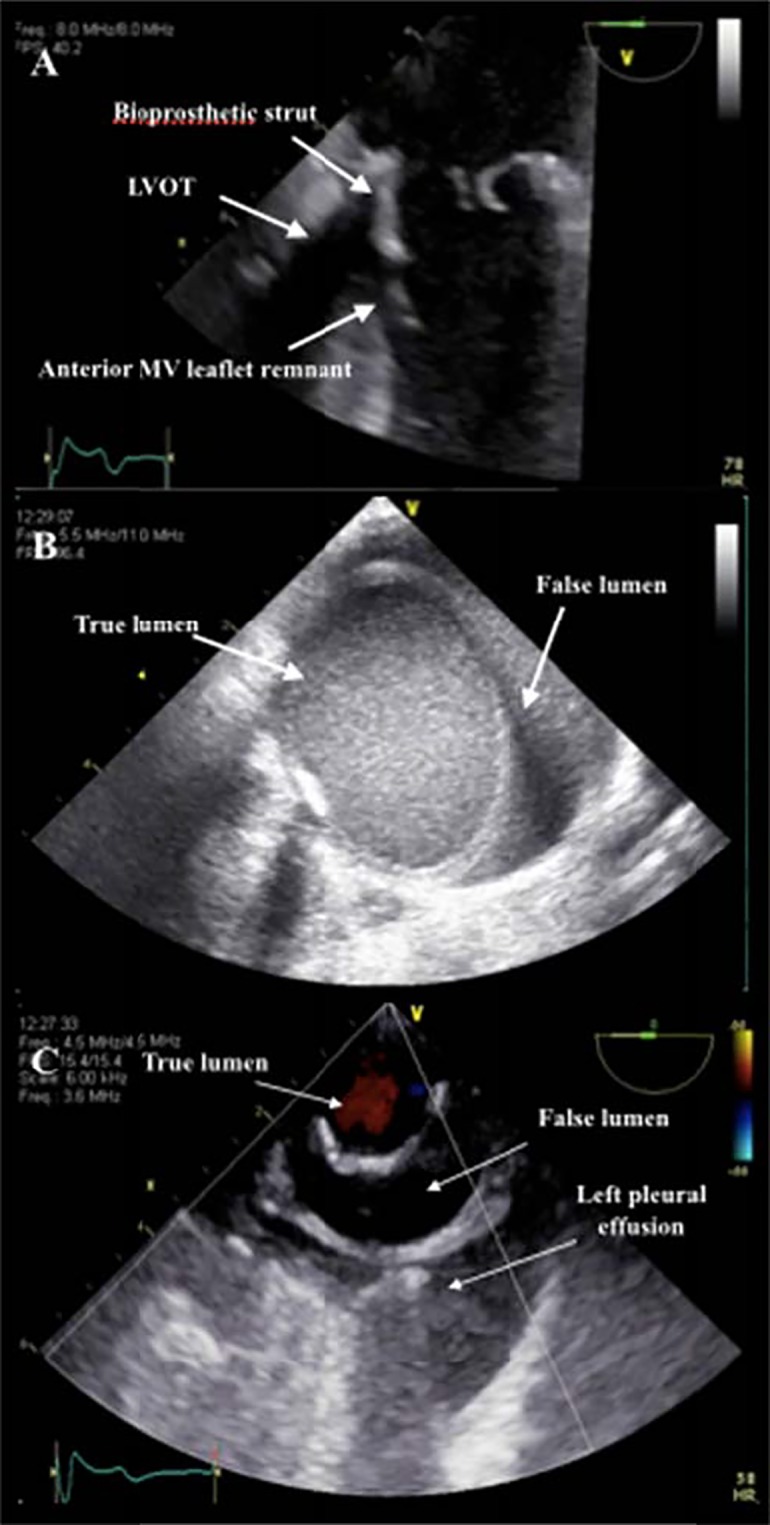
(A) Mid esophageal five-chamber view demonstrating LVOT obstruction from a bioprosthesis strut and anterior leaflet remnants. (B) Epiaortic ultrasound showing dissection of the ascending aorta. (C) Short axis view of the descending aorta with colour-flow Doppler to identify the true lumen of the aortic dissection. LVOT, left ventricular outflow tract; MV, mitral valve.
